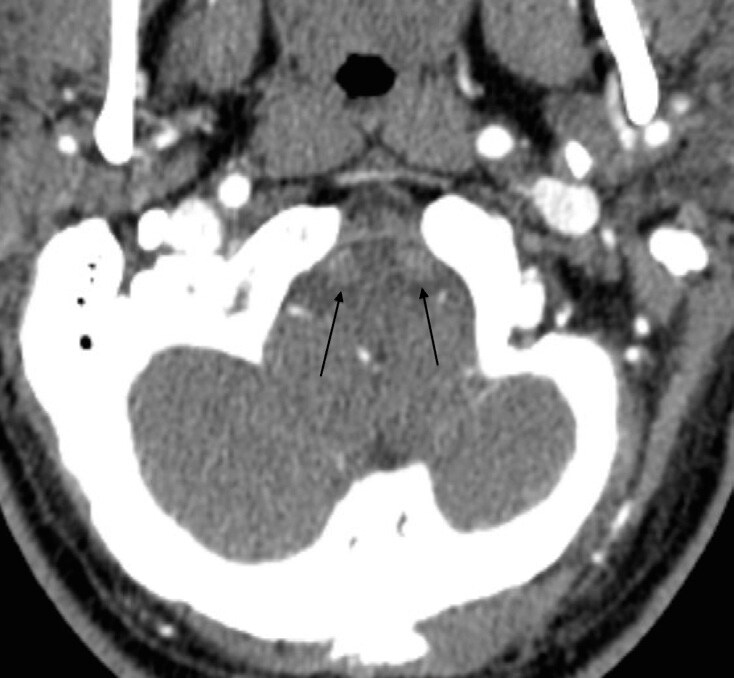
Axial computed tomography image showing decreased vertebral artery flow bilaterally at the level of the cerebellum.
